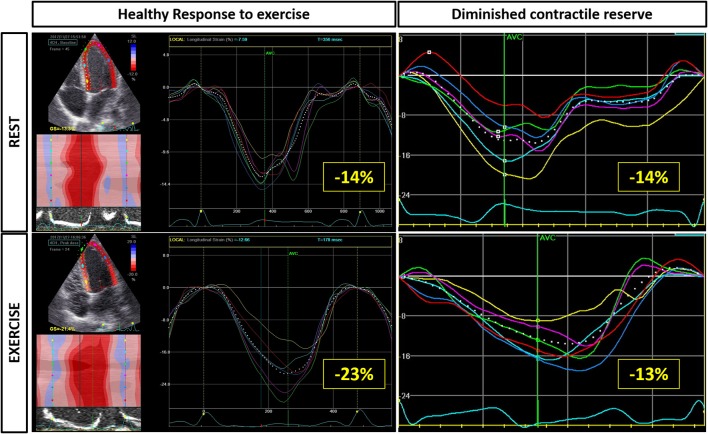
Deformation imaging with exercise can provide insights into subtle cardiac dysfunction. In this example, resting longitudinal strain assessed by 2D speckle tracking echocardiography is reduced in both patients. However, in the healthy heart strain increases from −14 to −23% but in the heart with abnormal contractility the strain does not augment with exercise. Furthermore, note that in the abnormal heart, the peak strain becomes progressively more delayed.
